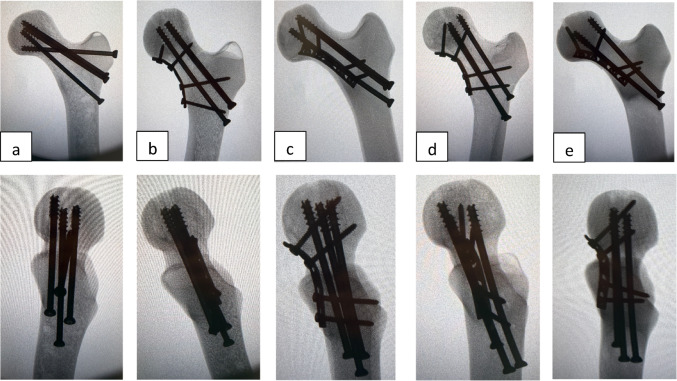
Anteroposterior (top row) and lateral (bottom row) radiographs of the fixation constructs: a Inverted Triangle with Pauwels screw, b Medial Buttress Plate with Inverted Triangle, c Anteromedial Support Plate with Inverted Triangle, d Medial Buttress Plate with L-Configuration, and e Anteromedial Support Plate with L-Configuration
